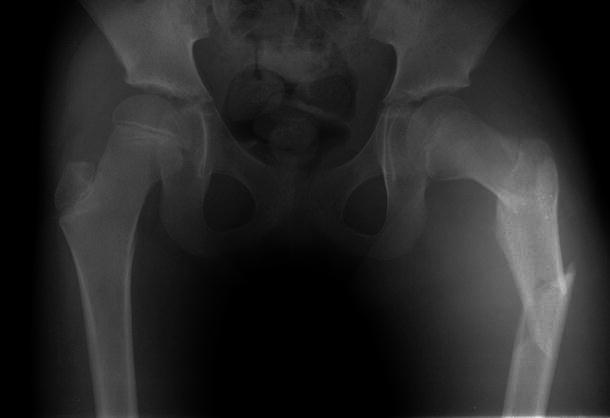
Eight-year-old girl: anteroposterior radiographs reveal a cervicotrochanteric fracture (type III in Delbet’s classification; AO classification type II) with a subtrochanteric fracture of the left femur
